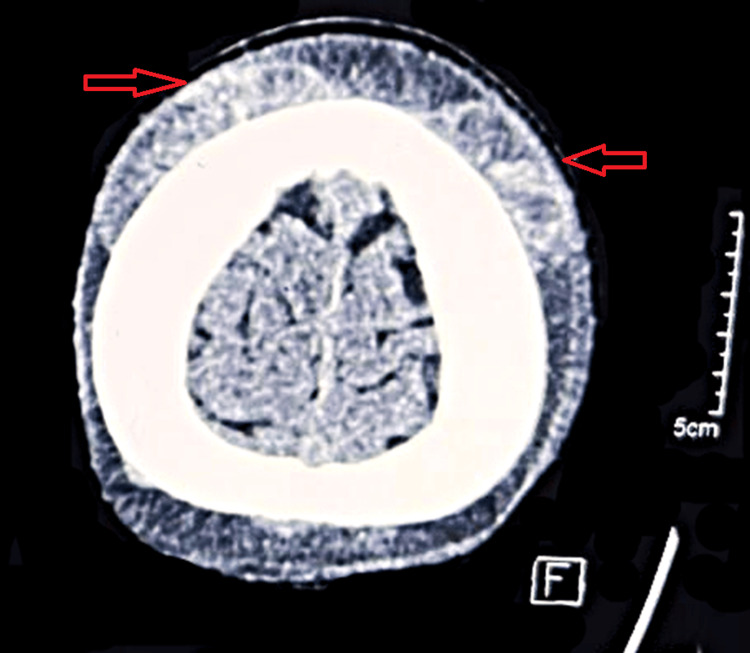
A non-contrast CT of the head showing the subgaleal hematoma (arrows) Non-contrast axial CT of the head showing the extracalvarial hyperdense region of hemorrhagic attenuation of 45 HU in subgaleal space of the bilateral frontoparietal region, suggestive of subgaleal hematoma.
